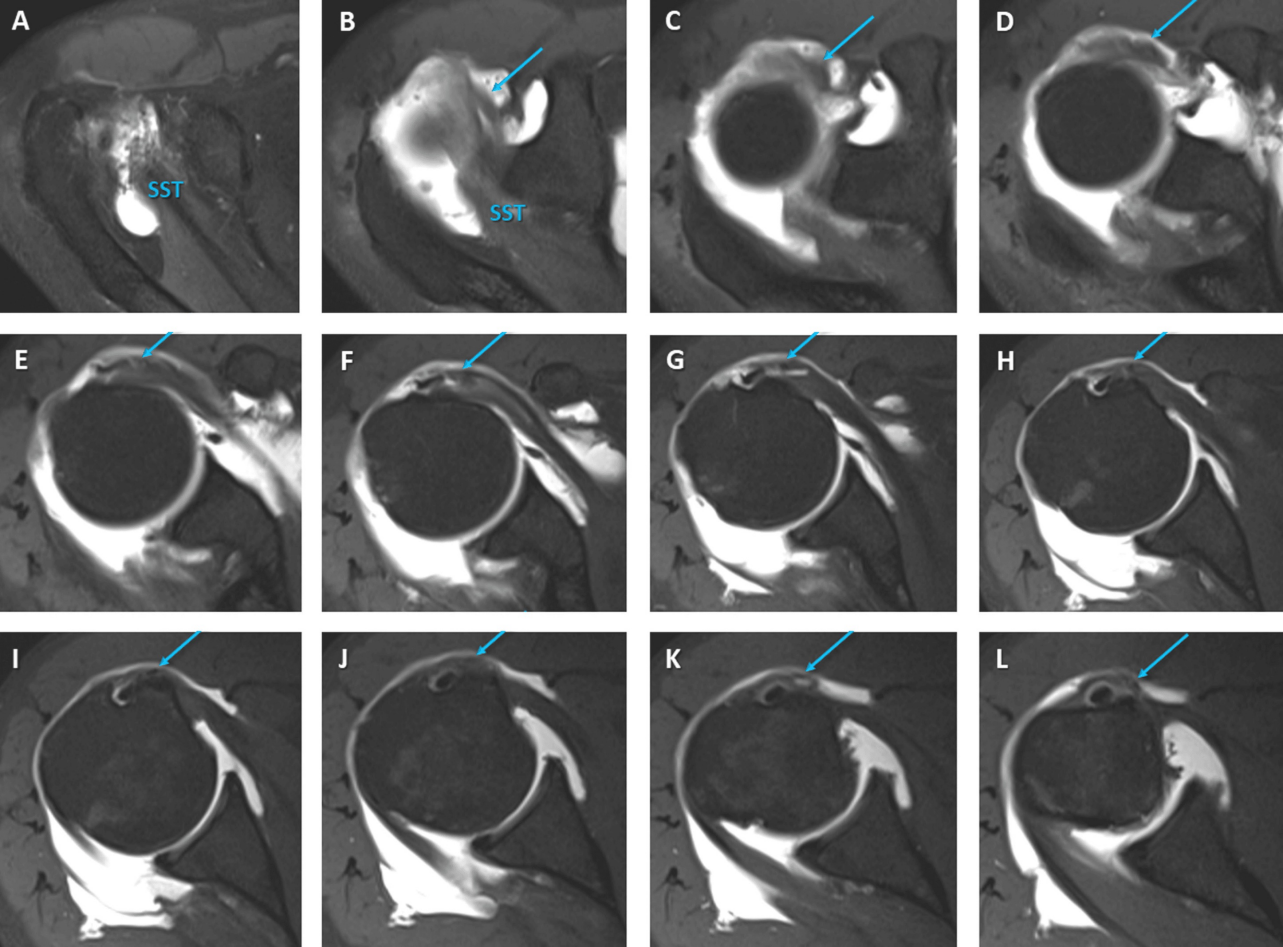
Consecutive transverse plane turbo spin echo (TSE) T1-weighted MR arthrograms It shows almost complete tearing of the supraspinatus (SST) and infraspinatus tendons, partial tearing of the subscapular tendon, and aponeurotic enlargement of the supraspinatus tendon (blue arrows).
